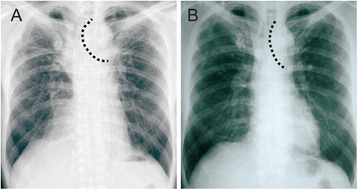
Chest radiograph taken at the a first visit and b 4 years before. The *dotted line* demarcates the left margin of the trachea
